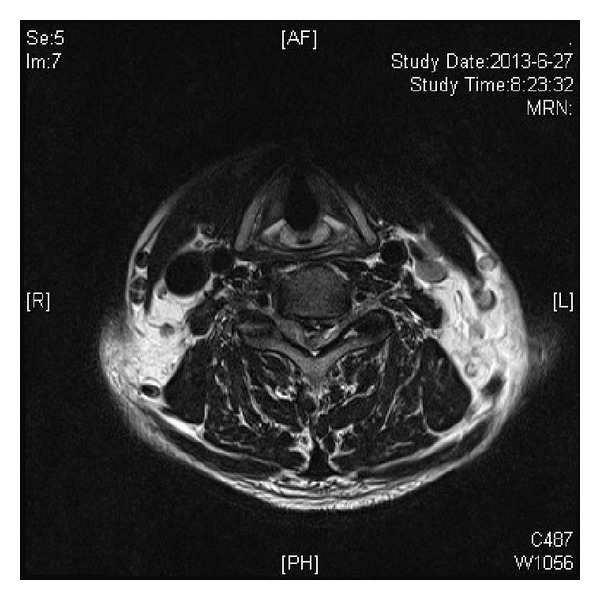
T2-weighted axial magnetic resonance image. The spinal cord is markedly compressed by a large disc herniation-like mass on the left side, with lateral displacement of the cord to the right. Zones of CSF signal intensity material (“halo”) are noted adjacent to the mass.
